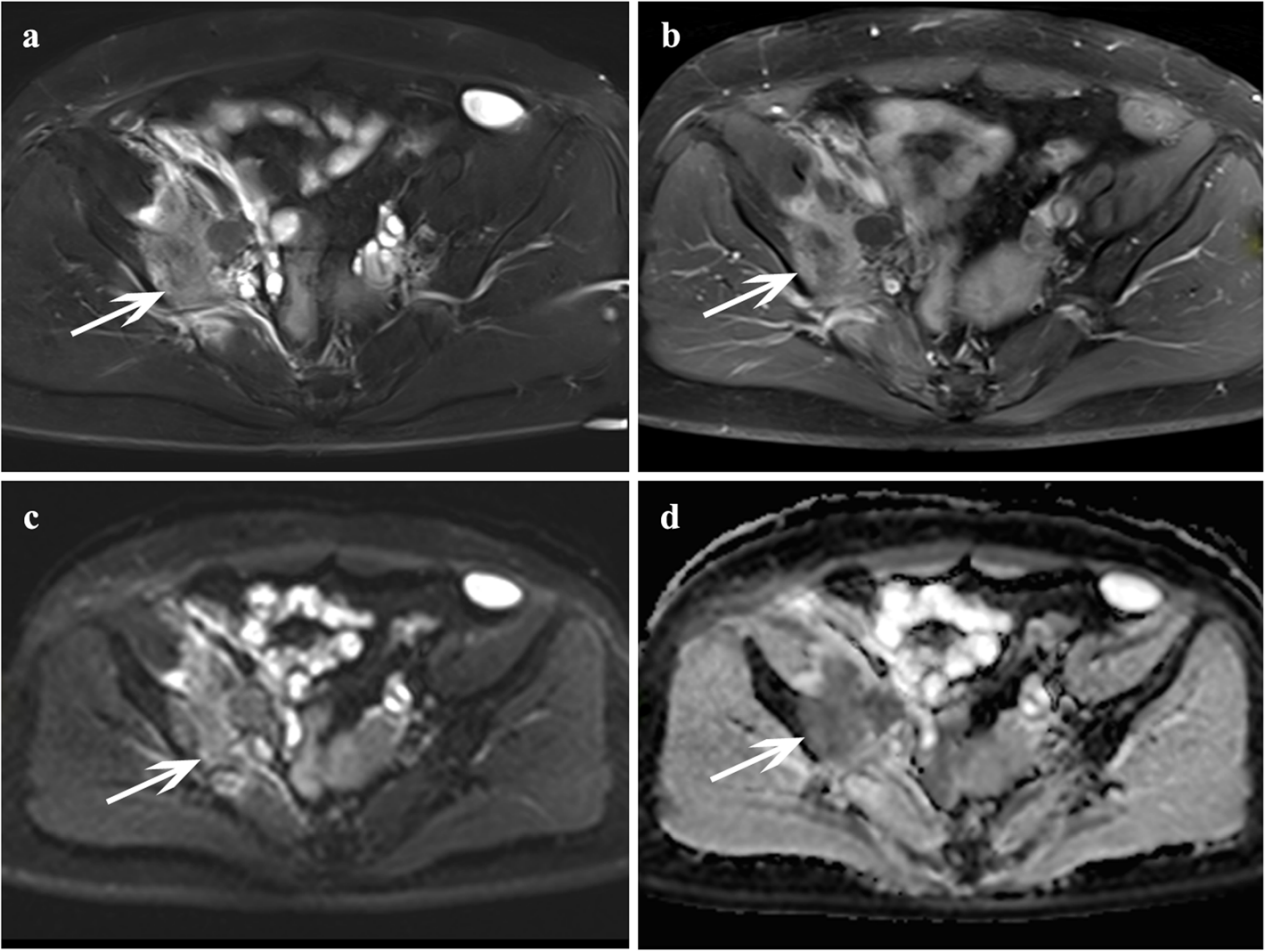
A 44-year-old female with cervical cancer after radiotherapy that was diagnosed with bone metastasis in the right ilium (white arrow). Axial FS T2-weighted image (a) showed a moderate hyperintensity solid mass in right ilium; the lesion showed contrast enhancement on axial T1-weighted image post-contrast, hyperintensity on DWI (c), and hypointensity on ADC map (d)
